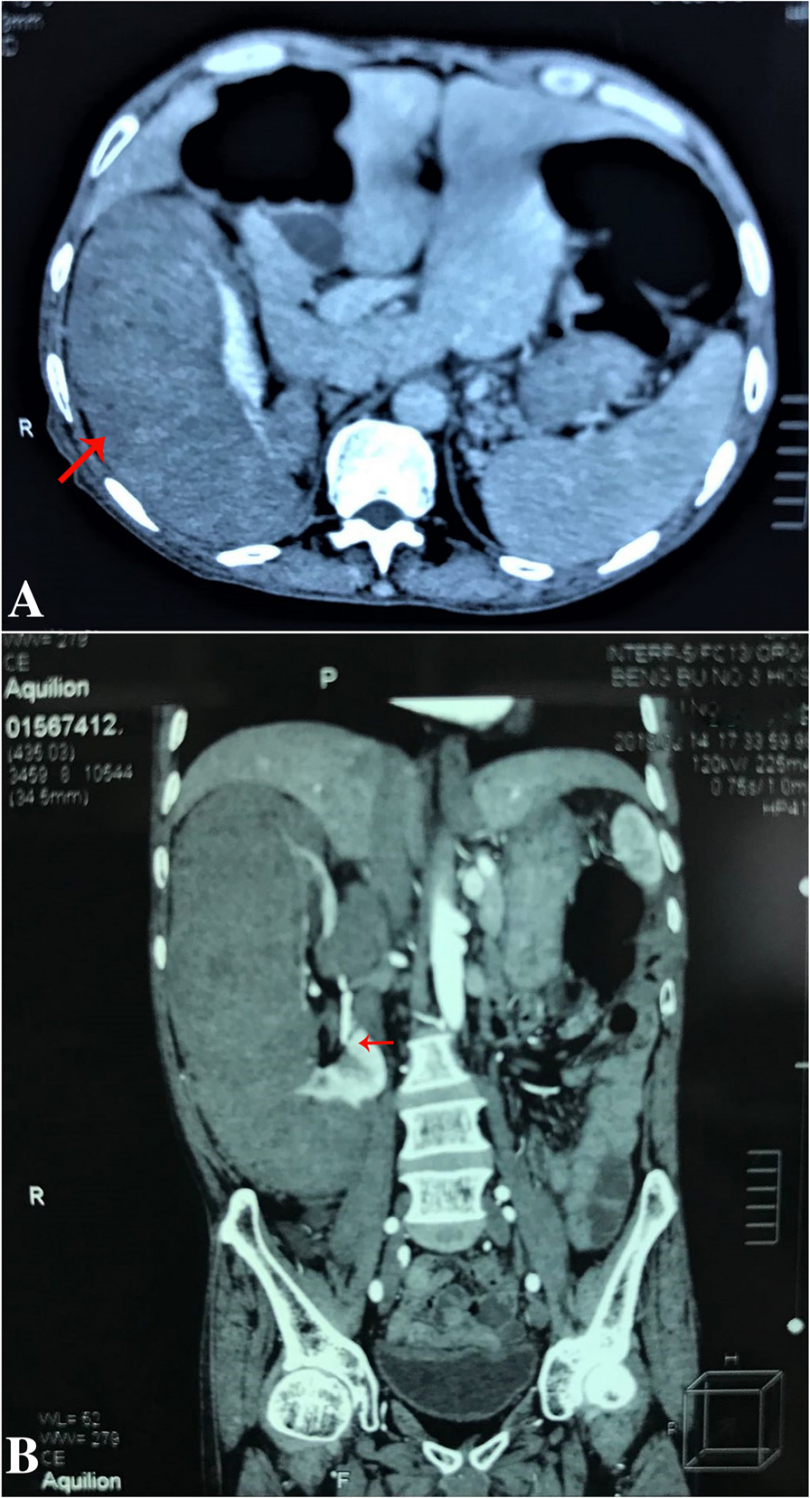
a CT scan demonstrates a large solid mass of the right kidney about 20 cm in diameter (arrow); b Renal arteriography demonstrated a hypovascular tumor and compressed deformity of pelvis of the right kidney (arrow)
